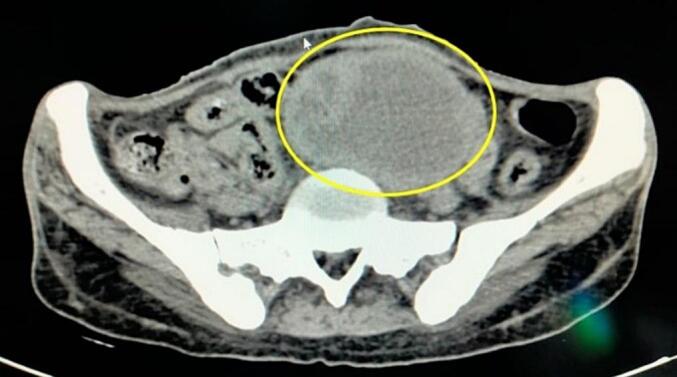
CT-Scan abdomen. CT imaging demonstrated a large left adnexal thick-walled, multiloculated, incomplete septations of 12 × 10 × 11cm, suggestive of a tubo-ovarian abscess or neoplastic lesion.
